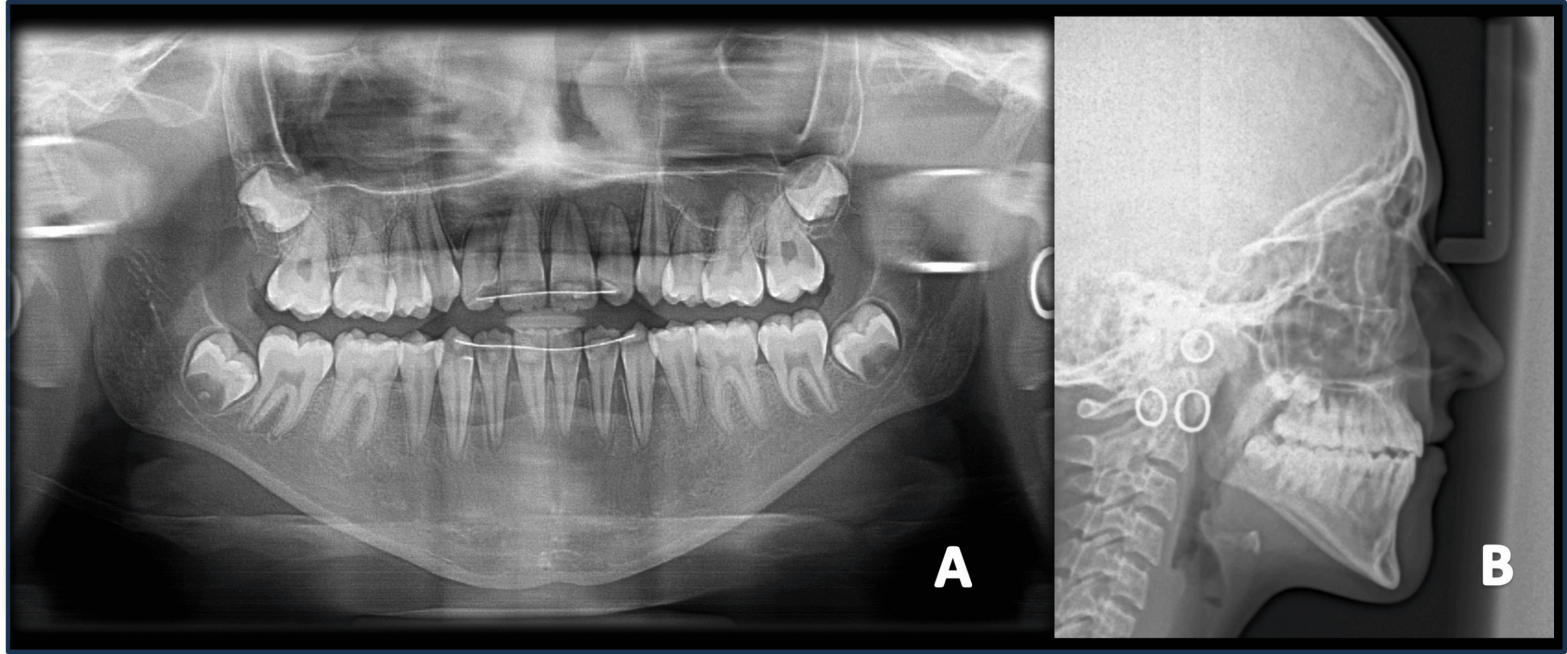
Post-treatment radiographs A) Panoramic view; B) Lateral cephalogram
